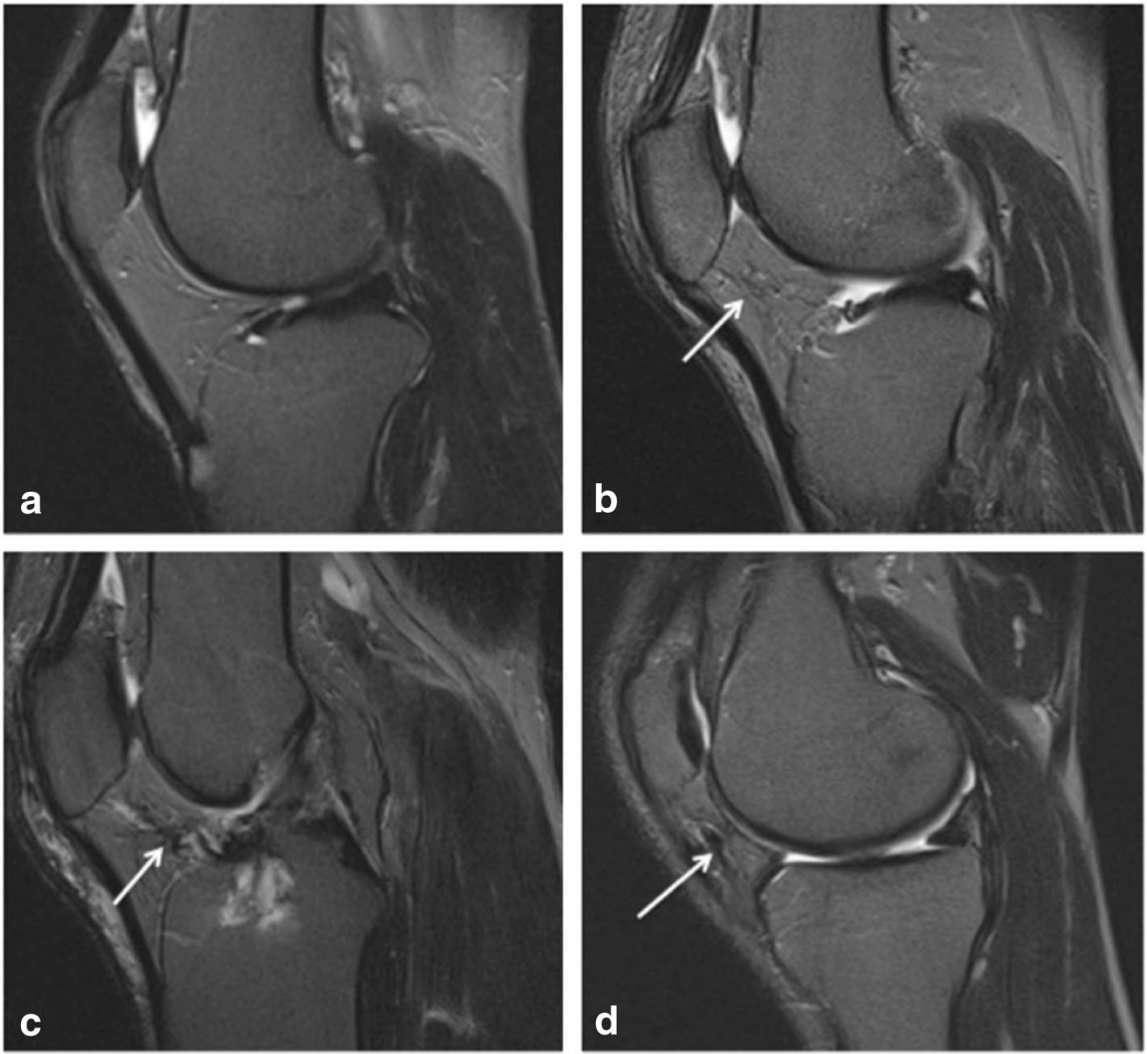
Hypointense signals on sagittal T2-weighted images with fat saturation. a Normal IPFP; b grade 1 hypointense signals of IPFP (*arrow*); c grade 2 hypointense signals of IPFP (*arrow*); d grade 3 hypointense signals of IPFP (*arrow*)
